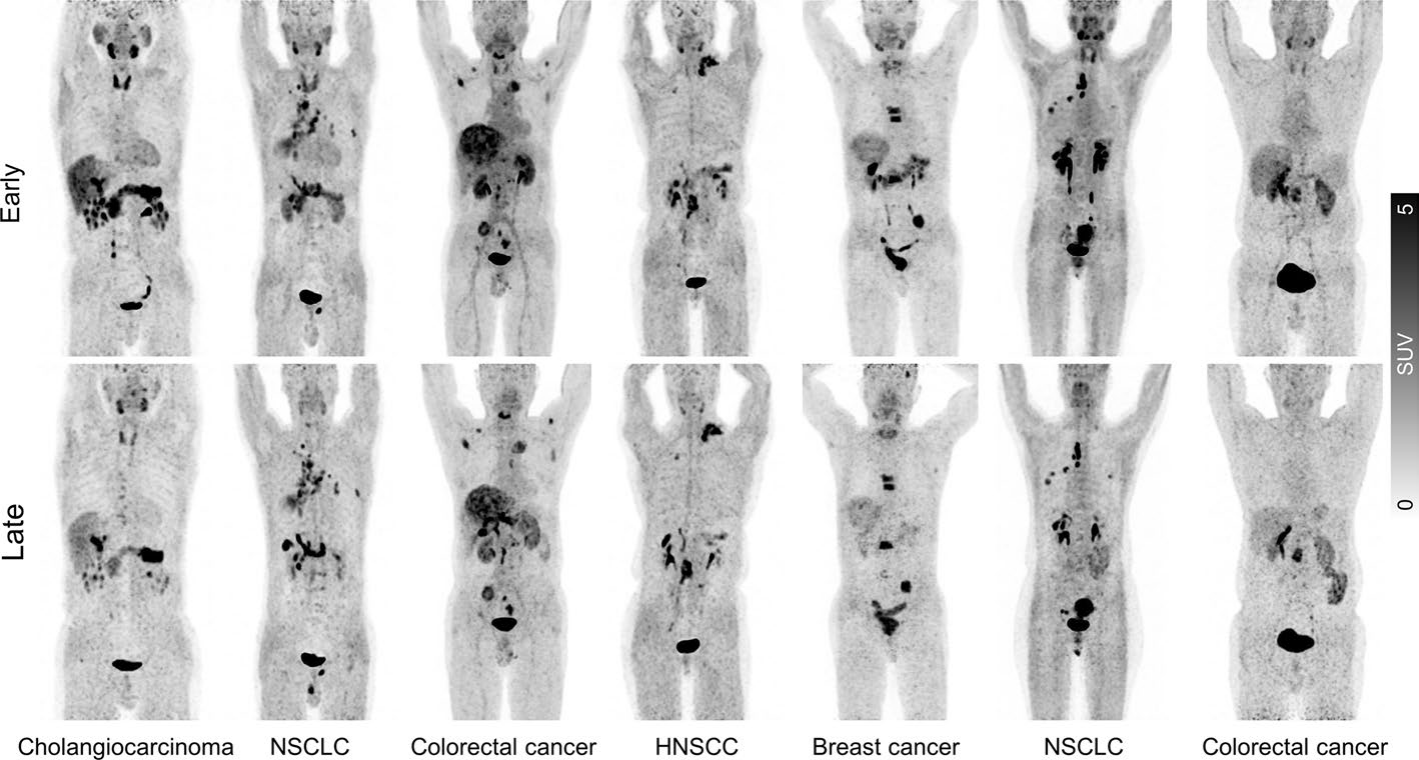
Maximum-intensity-projection images of [^18^F]FAPI-42 PET/CT at early and late timepoint in different types of cancer. NSCLC = Non-small cell lung cancer; HNSCC = Head and neck squamous cell carcinoma
